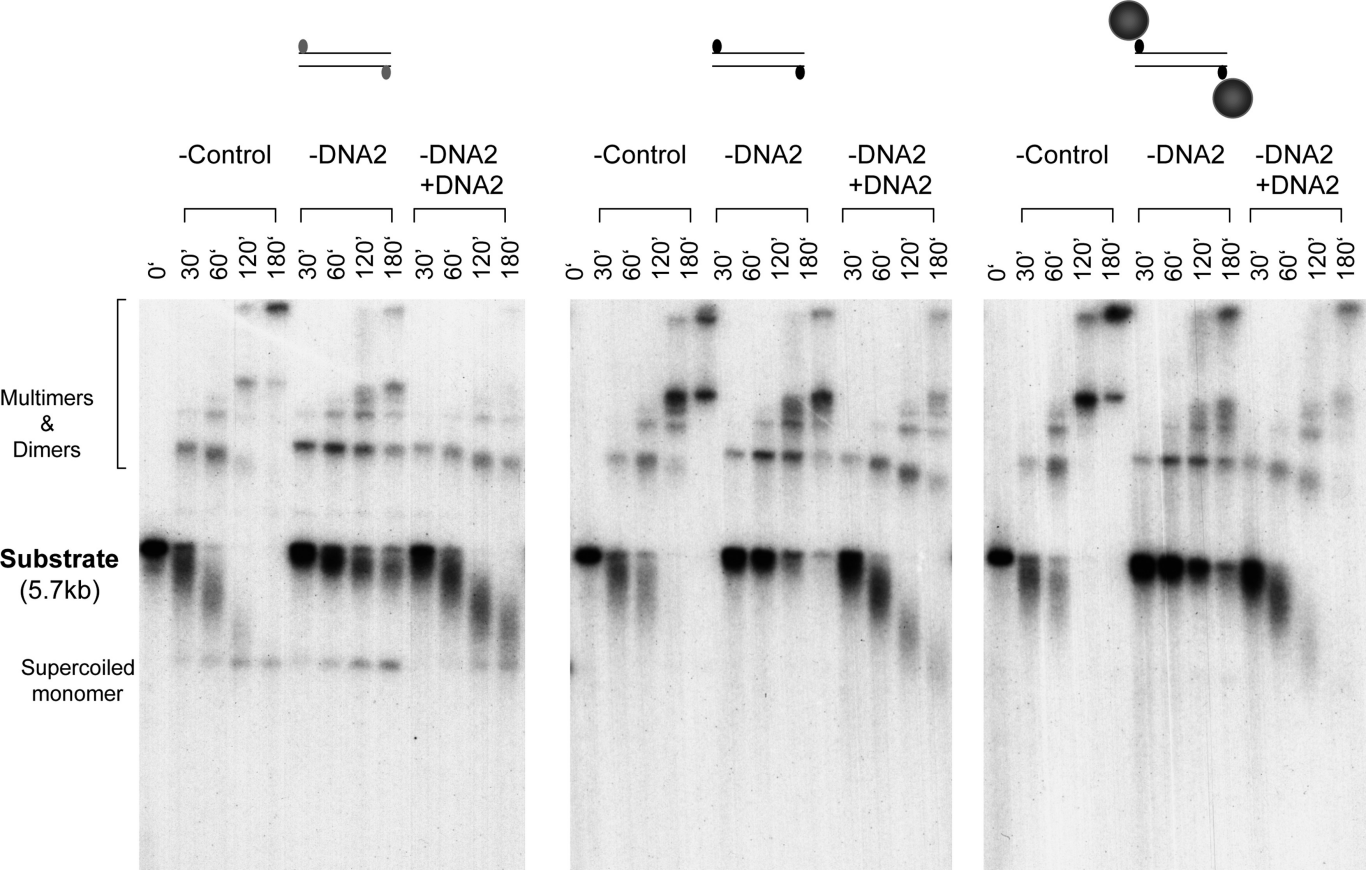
Rescue of the DNA2 depletion defect by the purified DNA2 protein. DNA substrates bearing different types of ends were incubated with the DNA2-depleted extracts supplemented with either buffer or DNA2 protein. Samples were treated with SDS-EDTA-Proteinase K, and separated on an 1% TAE-agarose gel. The gel was dried and exposed to X-ray film.
